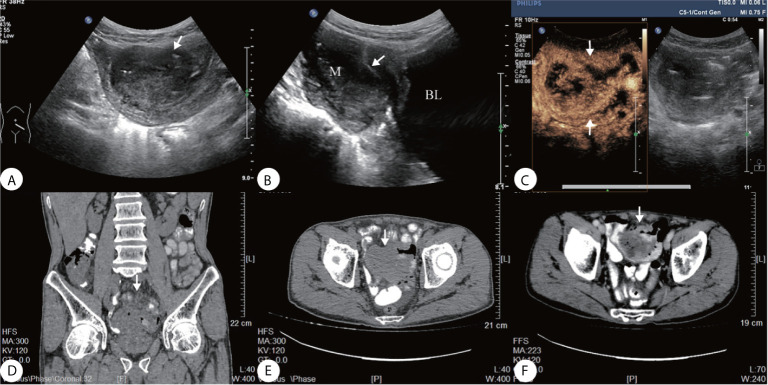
(A) Ultrasound showed a well-defined heterogeneous hypoechoic mass on the left lower abdomen, (B) the mass close contact with the bladder wall (arrow); (C) Contrast-enhanced ultrasonography (CEUS) presented irregular necrotic areas, heterogeneous enhancement in the arterial phase with quick wash-out (54 s); (D–F) Abdominal CT showed a hypodense solid lesion in the wall of an ileal loop, with areas of necrosis within (arrow).
